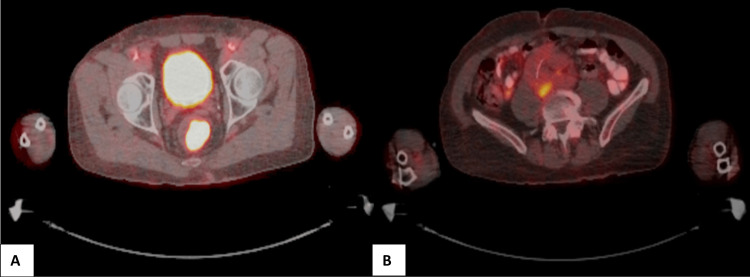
PET scan images The following PET scan images demonstrate the intensely FDG avid lesion in the mid-rectum corresponding to the known primary (A) and the low-density retrocaval mass suspicious for a metastatic lymph node (B). PET: positron emission tomography, FDG: fluorodeoxyglucose
